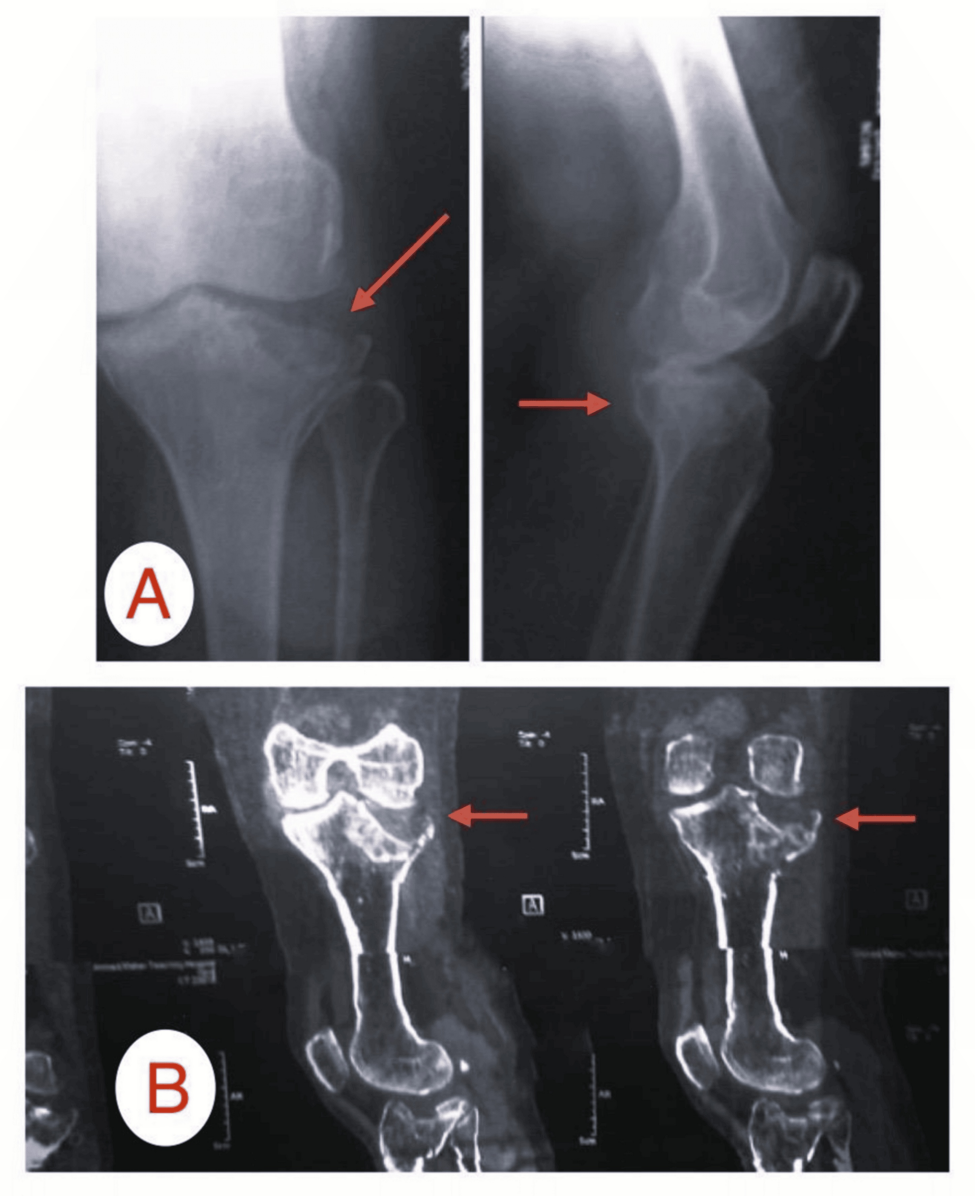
Preoperative images of Patient A: (A) X-ray showing a depressed fracture of the tibial plateau, (B) CT scan showing a depressed lateral tibial plateau fracture
